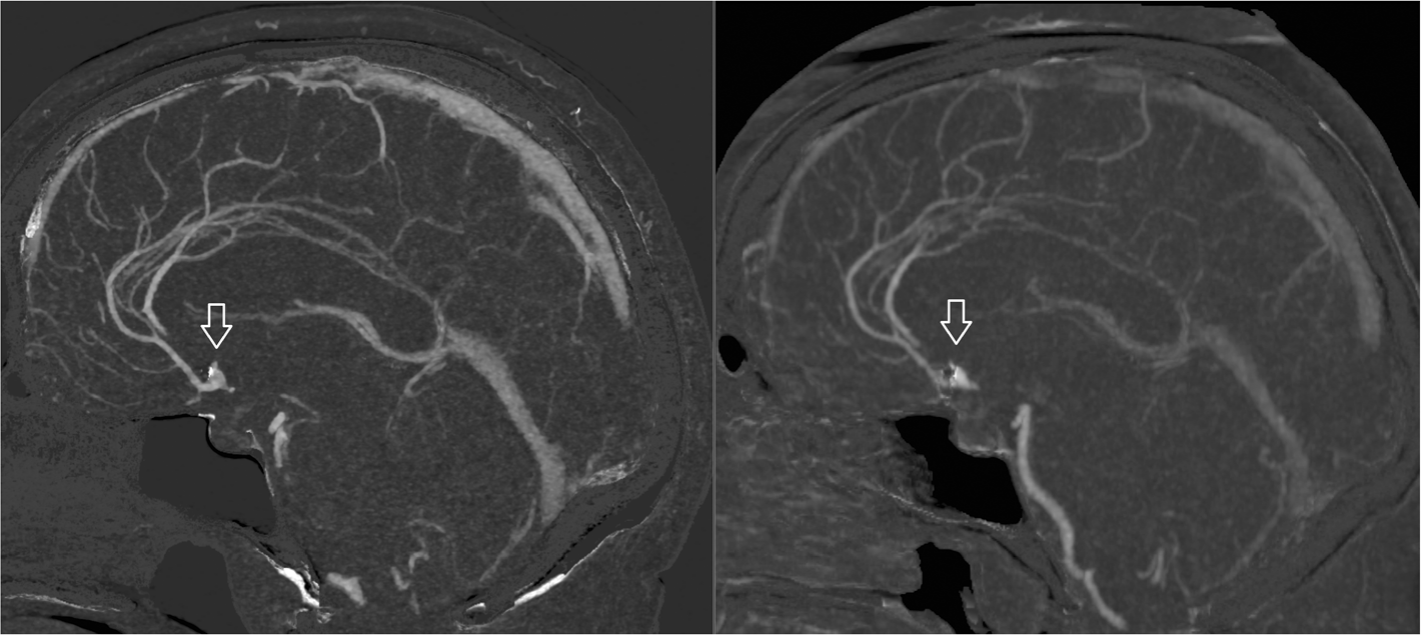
Sixty-nine-year-old female with surgical clip-treated anterior communicating aneurysm. Follow-up with subtraction CTA demonstrates a remnant of the aneurysm (arrows). Image quality of subtraction CTA is superior on the UHR system (left image) as compared to subtraction CTA on a conventional CT system (right image) due to increased spatial resolution (0.25 × 0.25 mm versus 0.5 × 0.5 mm)
